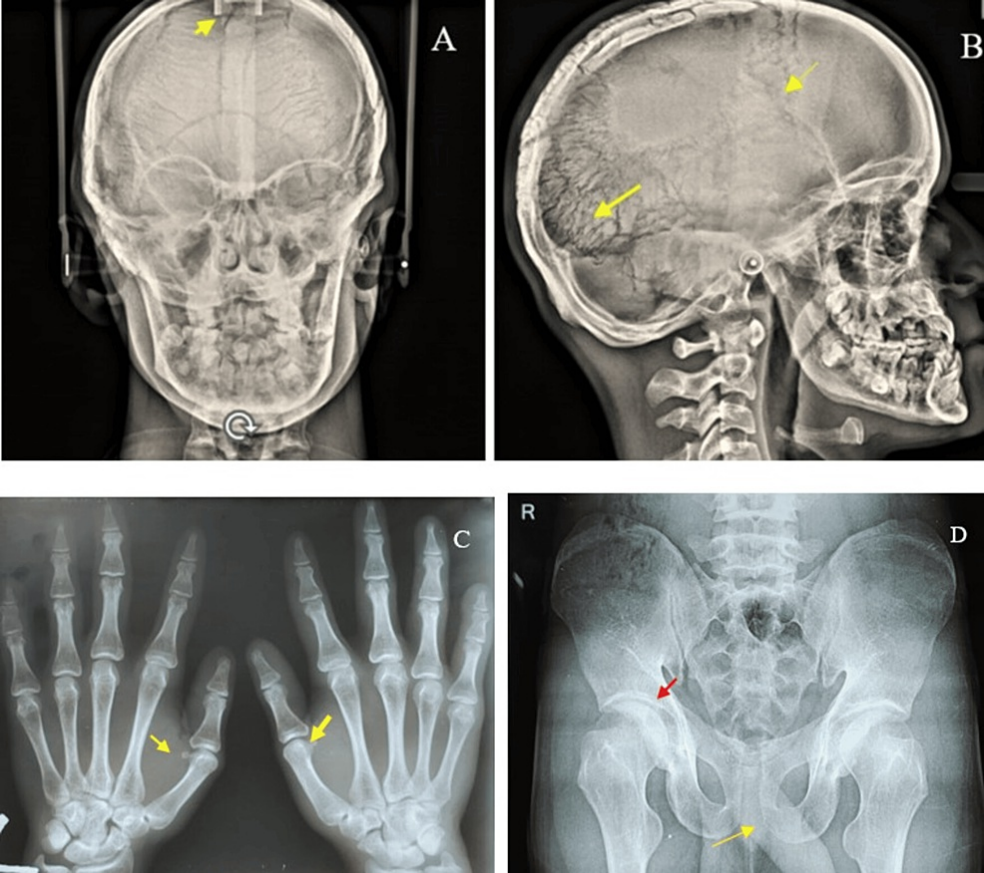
(A) Anteroposterior skull radiograph revealing a lack of closure of sutures on the skull, resulting in open fontanelles (yellow arrow). (B) Lateral skull radiograph revealing increased sutural markings on the skull and nonunion of the cranial bone (yellow arrow). (C) Hand-wrist radiograph revealing metaphyseal deformities of the first metacarpophalangeal bone in both the right and left forearms (yellow arrow). (D) Pelvic X-ray revealing coxa valga (red arrow) and increased pubic symphysis diastasis (yellow arrow)
